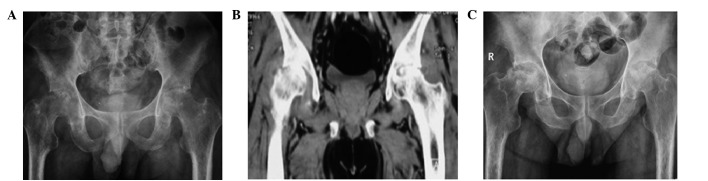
X-ray of the pelvis reveals bilateral femoral head collapse. (A) Widespread changes to the bone mass of the pelvis and bilateral femurs. The bilateral femoral head is flat, with an uneven bone density. A region of multiple small cystic bone destruction under the articular surface is visible, with a rough articular surface. The joint space is narrowed, the upper edge of the acetabular is hardened and the right lateral femoral head has shifted slightly outwards to the eutopic left hip. (B) Computed tomography shows ischemic necrosis of the bilateral femoral head and effusion of the right hip joint. The surrounding tissue of the bilateral hip is swollen. The load-bearing surface joint space of the right hip joint has narrowed, whereas the non-load-bearing surface joint space has widened. The gap in the left hip is uniformly narrower, the head shape of the bilateral femor is irregularly flat, the surface is rough, multiple bone density-reducing regions are visible in the bone mass and the surrounding soft tissue is swollen. (C) Pelvic X-ray after six months of treatment reveals that the collapse of the bilateral femoral head of the patient is more severe than that six months earlier. The bilateral femoral head was flatter, which indicates that the ANFH of the patient had developed gradually following the injection of heroin.
